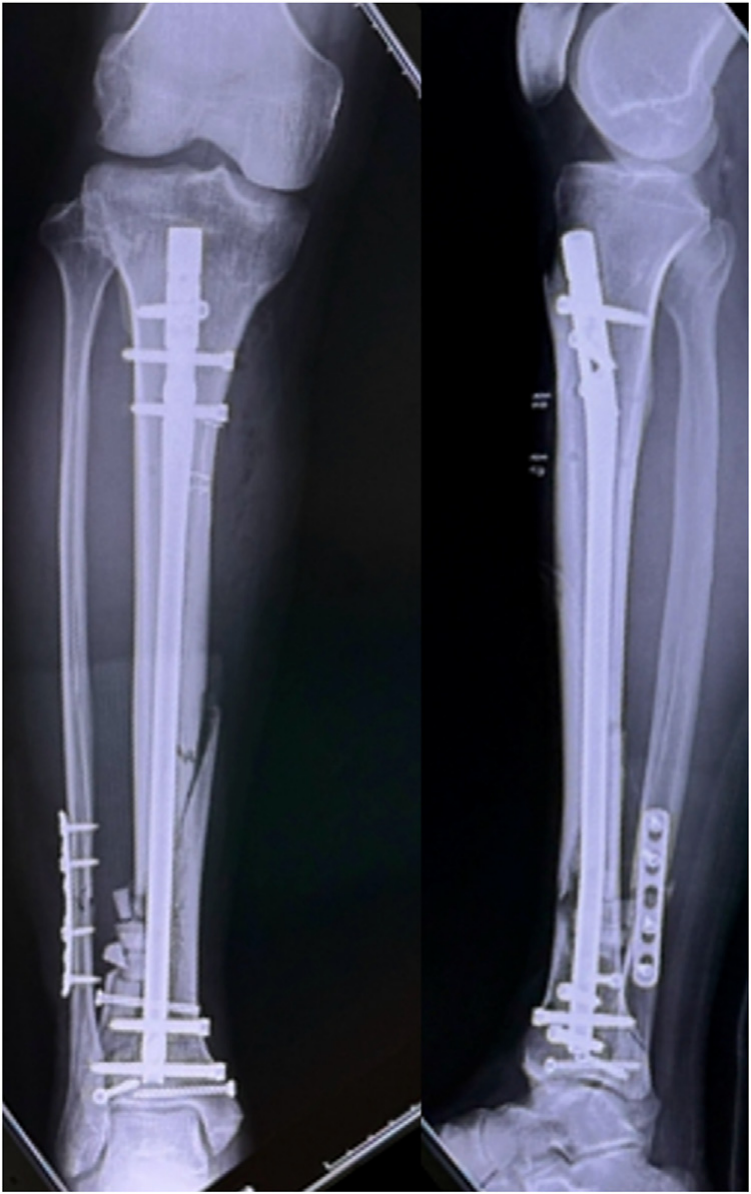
Plain radiograph after osteosynthesis using intramedullary nail and plate.
